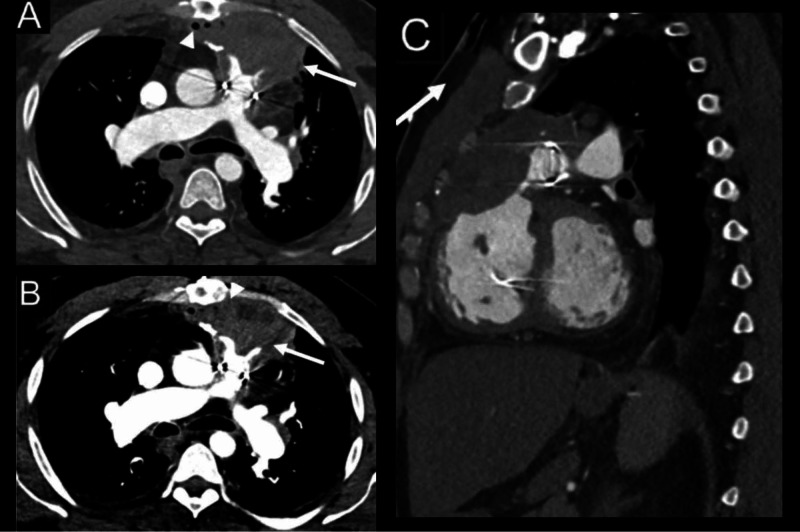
CT pulmonary angiogram of the chest showing the mediastinal abscess with a superimposed hematoma. Axial CT pulmonary angiogram shows a marked increase in size of the anterior mediastinal lesion (A, arrow), which causes encroachment and mass effect on the pulmonary artery outflow tract without active contrast extravasation. Air foci in the anterior mediastinum represent ongoing infection (A, arrowhead). On narrow window settings, hyperdense hematoma is seen abutting the pulmonary outflow tract (B, arrow), in contrast to less dense anterior abscess (B, arrowhead). Expanding hematoma extends to compress the right ventricular outflow tract (C, arrow).
